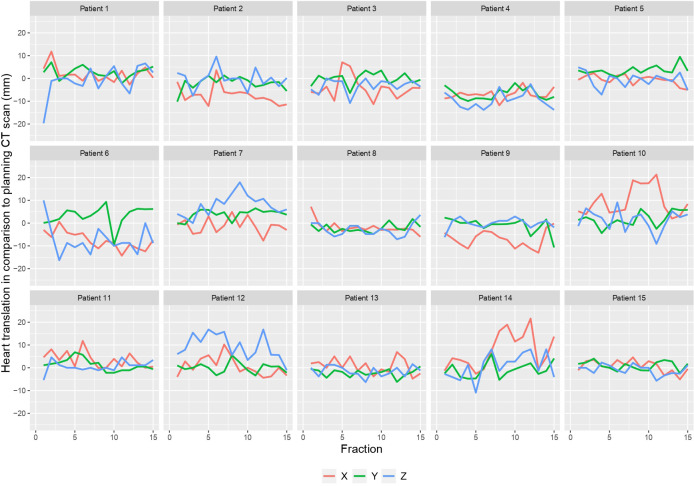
Heart translation per patient in each axis (X, Y, Z) in comparison to the planning CT scan.
